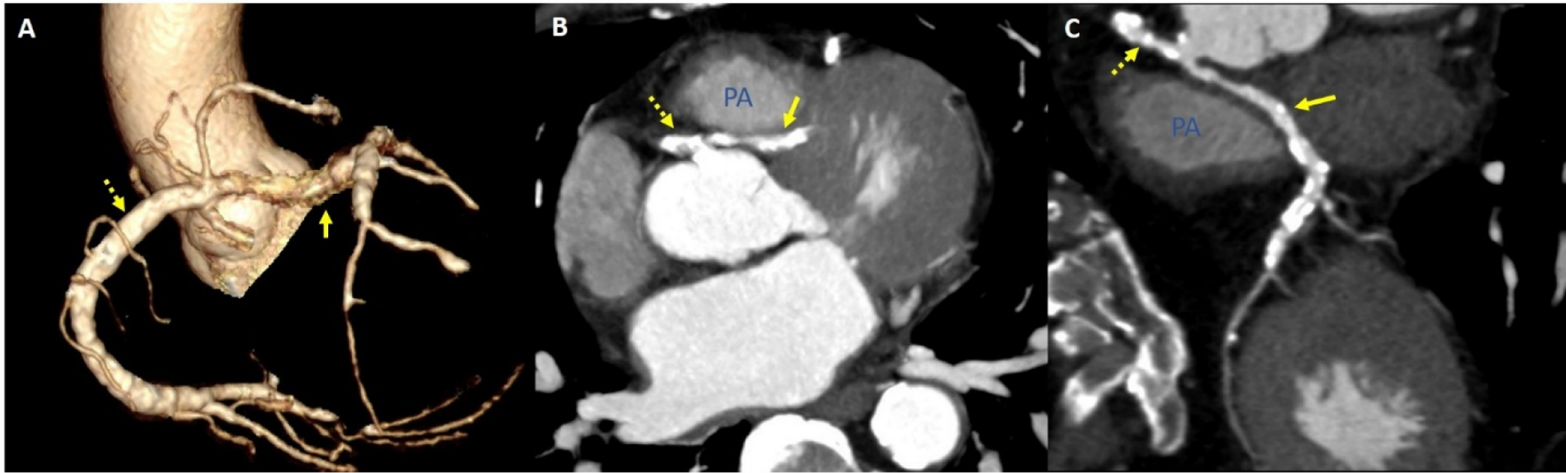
Coronary computed tomography angiography assessment for anomalous aortic origin of a coronary artery. (A) Reconstructive image revealed “right” main bifurcation. (B,C) Coronary computed tomography angiography showed a common ostium for the left main coronary artery and the right coronary artery, characterized by an interarterial course.
